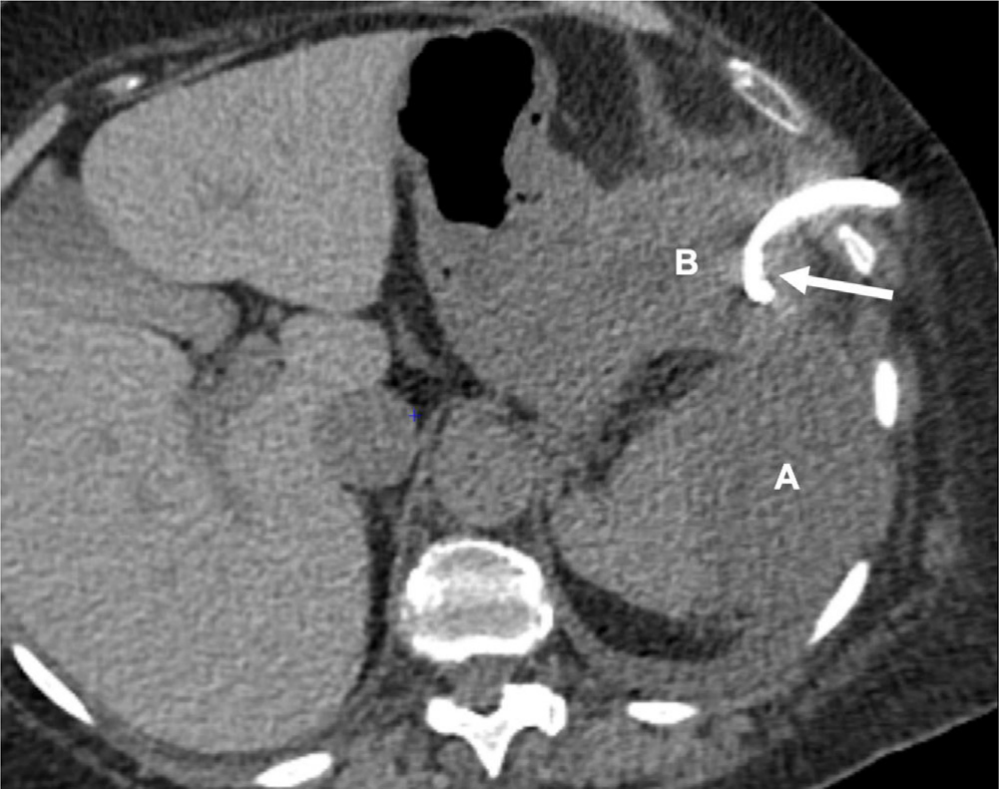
Non-contrast-enhanced CT. Axial MPR, performed after percutaneous drain (arrow) placement in the perisplenic hematic collection (B) fistulized into the splenic collection (A).
